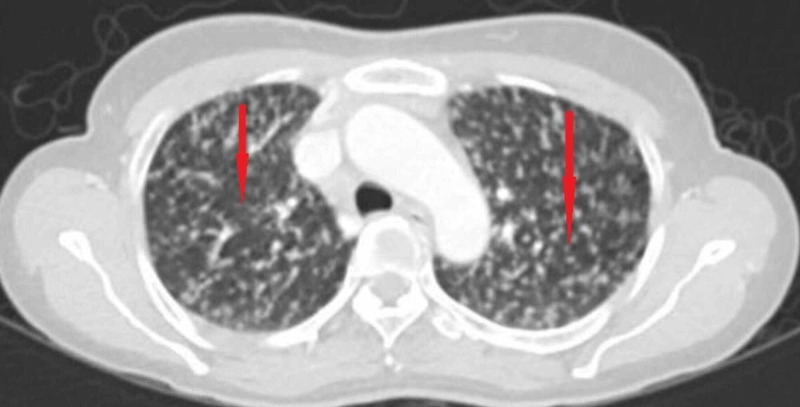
Chest CT scan Chest computerized tomography (CT) scan showing bilateral numerous miliary nodules, some are indicated by red arrows.
